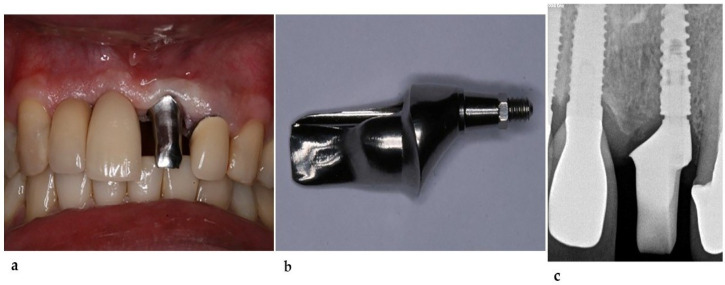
(a) Customized abutment positioned in mouth. (b) Customized abutment. (c) Radiographic aspect of the abutment over the implant, showing the component adaptation.
